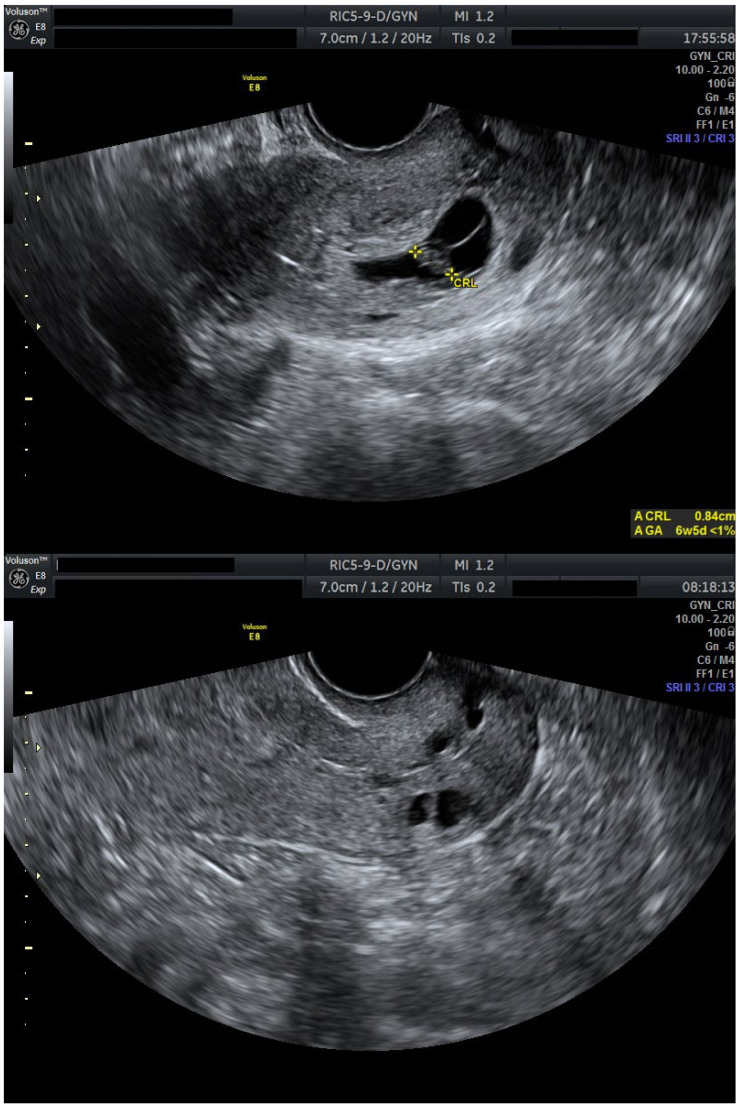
Cervical canal before and after suction curettage (Day 13). Transvaginal ultrasound evaluation: sagittal view through the cervical canal. A total of 24 h after initiation of misoprostol expulsion therapy. Impacted gestational sacs in the cervical canal before curettage and the empty cervical canal immediately after suction curettage. Patient information is anonymized.
